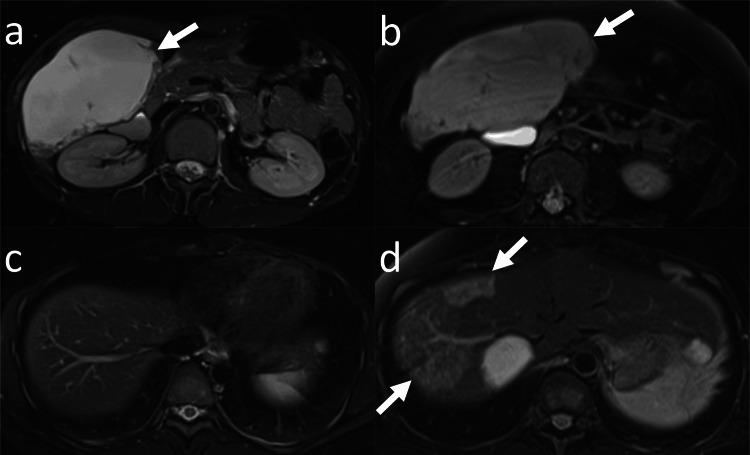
Enlargement of a hepatic hemangioma and hemangiomatosis in a 32-year-old woman (Case 2 in Table 1) (a) Axial fat-saturated T2-weighted magnetic resonance imaging (MRI) (baseline study) of the upper abdomen shows a hepatic hemangioma (arrow) with a diameter of 109 mm located in the right lobe of the liver. (b) Axial fat-saturated T2-weighted MRI (final study) of the upper abdomen obtained at the 138-month follow-up shows an enlargement of the hepatic hemangioma (arrows). The diameter of the hepatic hemangioma is 149 mm. (c) Axial fat-saturated T2-weighted MRI (baseline study) at the diaphragm shows no hepatic hemangiomatosis. (d) Axial fat-saturated T2-weighted MRI (final study) at the diaphragm shows innumerable small nodular high signal intensity (arrows), consistent with hepatic hemangiomatosis.
